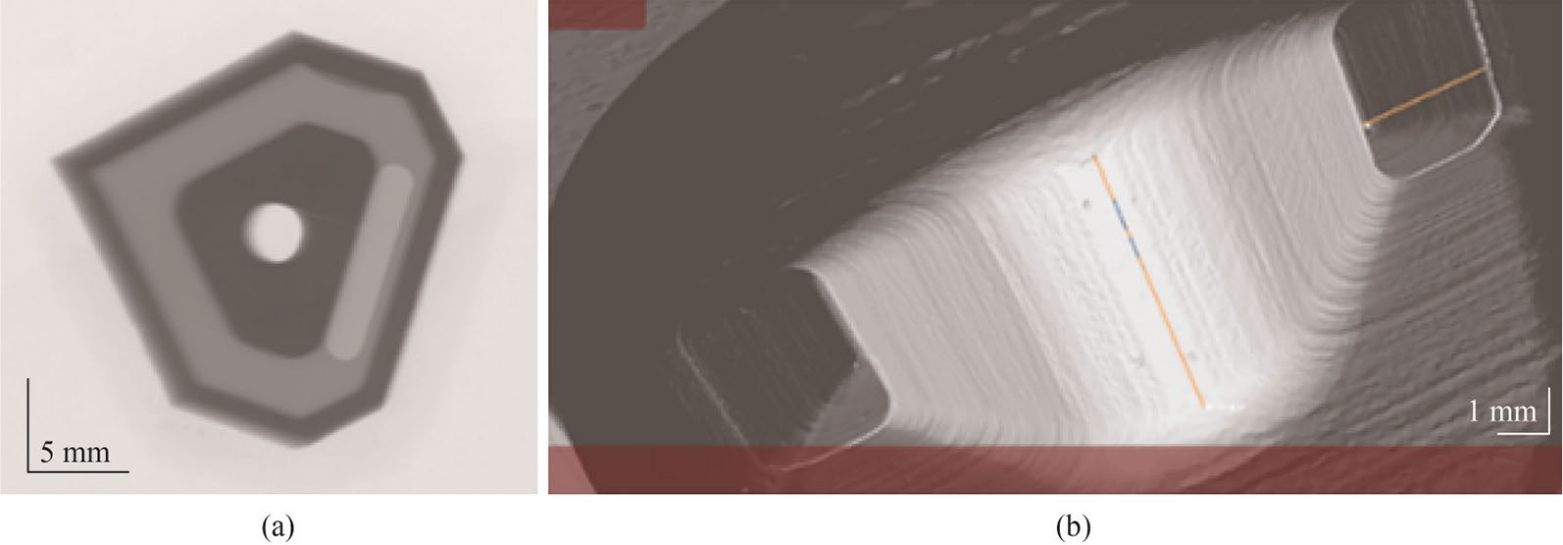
a X-ray, and b CT image of the internal channel
